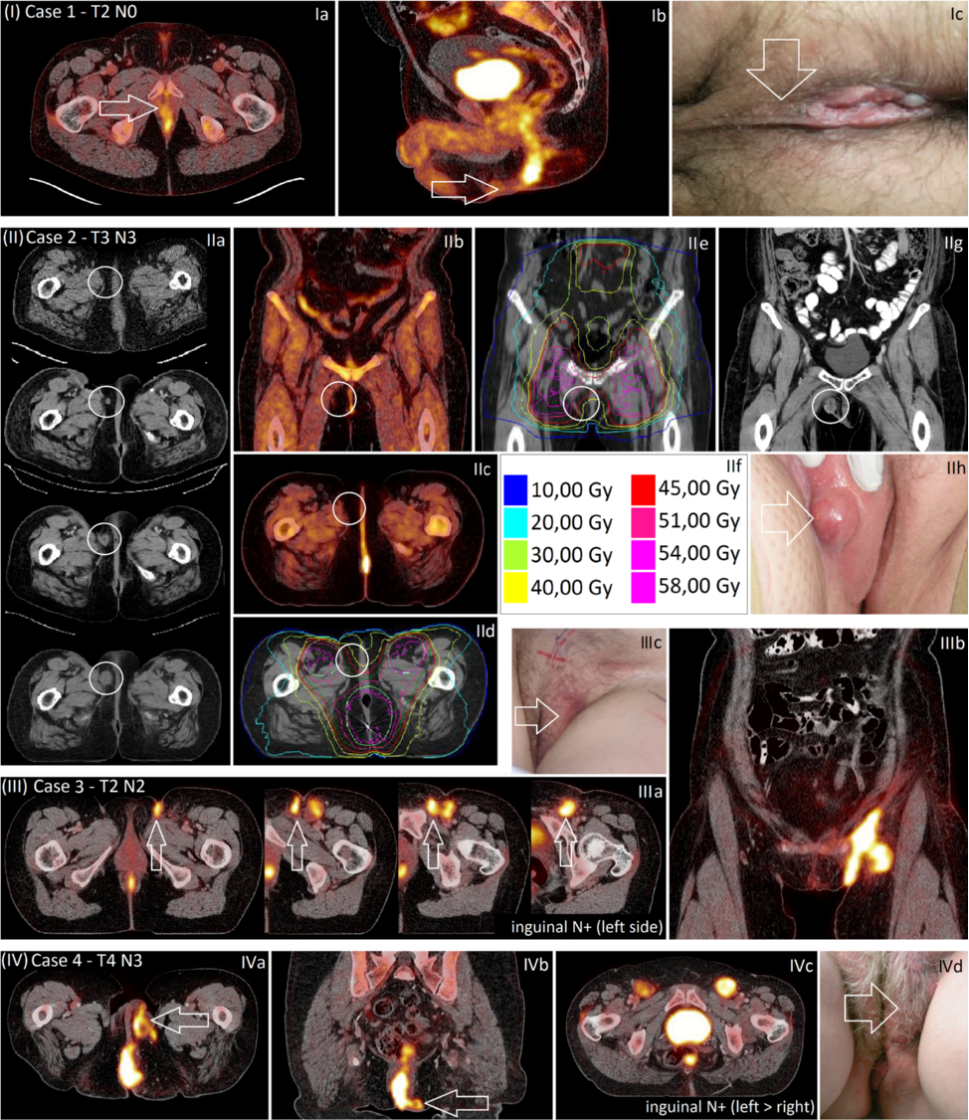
Direct genital infiltration and perigenital involvement and/or perivulvar relapse in anal carcinoma: PET/CT images and clinical manifestation. Legend: (I) Direct genital infiltration in N0/N3 anal cancer at time of first diagnosis (case 1) with PET/CT images in transversal and sagittal planes (Ia, b) and clinical photo (Ic); (II) Vulvar/perivulvar relapse 9 months after concurrent IMRT and chemotherapy of an inguinally N+ anal cancer (case 2) with the CT-sequence of the development of the relapse from time of first diagnosis until 9 months after radiochemotherapy (IIa), pretherapeutic PET/CT images in transversal and coronal planes (IIb, c), IMRT plans in transversal and coronal planes with isodose details (IId, e, f), CT image of the relapse in coronal plane and clinical photo at 9 months after therapy (IIg, h); (III) and (IV) Perigenital involvement related to lymphatic spread in inguinally N+ anal cancer at time of first diagnosis (case 3 and case 4) with PET/CT images in transversal and coronal planes (IIIa, b and IVa, b, c) and clinical photo (IIIc and IVd)
